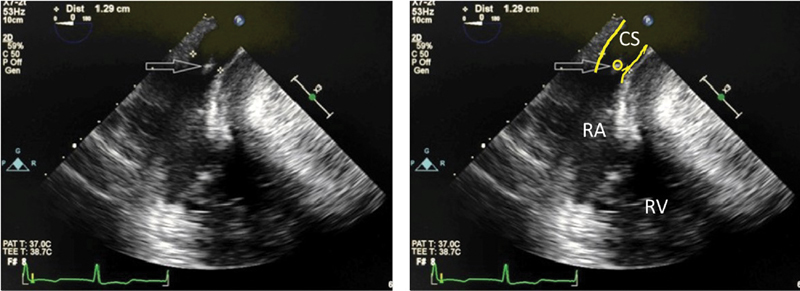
Intraoperative transesophageal echocardiogram (TEE) showing the pulmonary artery (PA) catheter (yellow circle) exiting the coronary sinus (yellow lines) into the right atrium. The coronary sinus diameter was 1.29 cm. CS, coronary sinus; RA, right atrium; RV, right ventricle.
